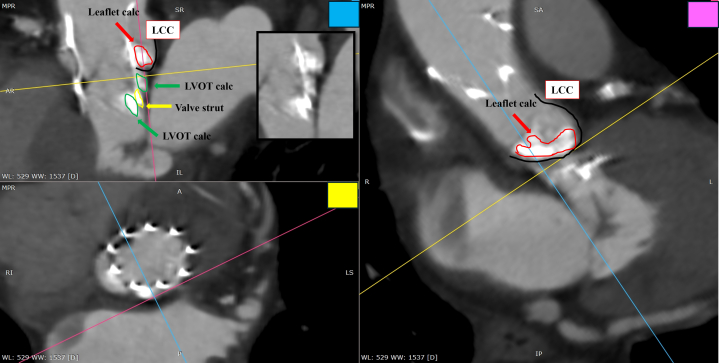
Postprocedural Multiplanar Reconstructed Computed Tomography The red arrows indicate leaflet calcification, the green arrows indicate LVOT calcification, and the yellow arrow indicates the strut at the valve's lower edge. calc = calcification; LCC = left coronary cusp; LVOT = left ventricular outflow tract.
